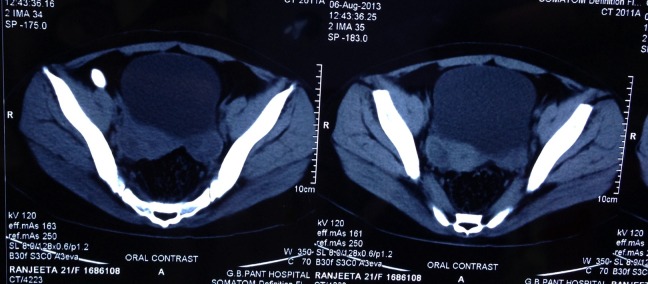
CT scan of the pelvis showing normal ovaries with the presence of only follicular cysts after 6 months of therapy.
